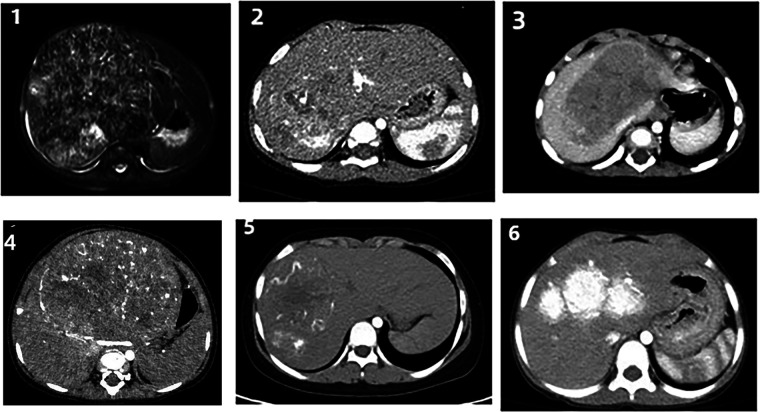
Figures 1–5 Contrast-enhanced CT images obtained at the time of diagnosis for patients 1–5, showing the presence and location of tumors in the liver. Figure 6 shows a post-interventional embolization image of patient 6, revealing successful arterial occlusion following selective arterial embolization before sugey.
